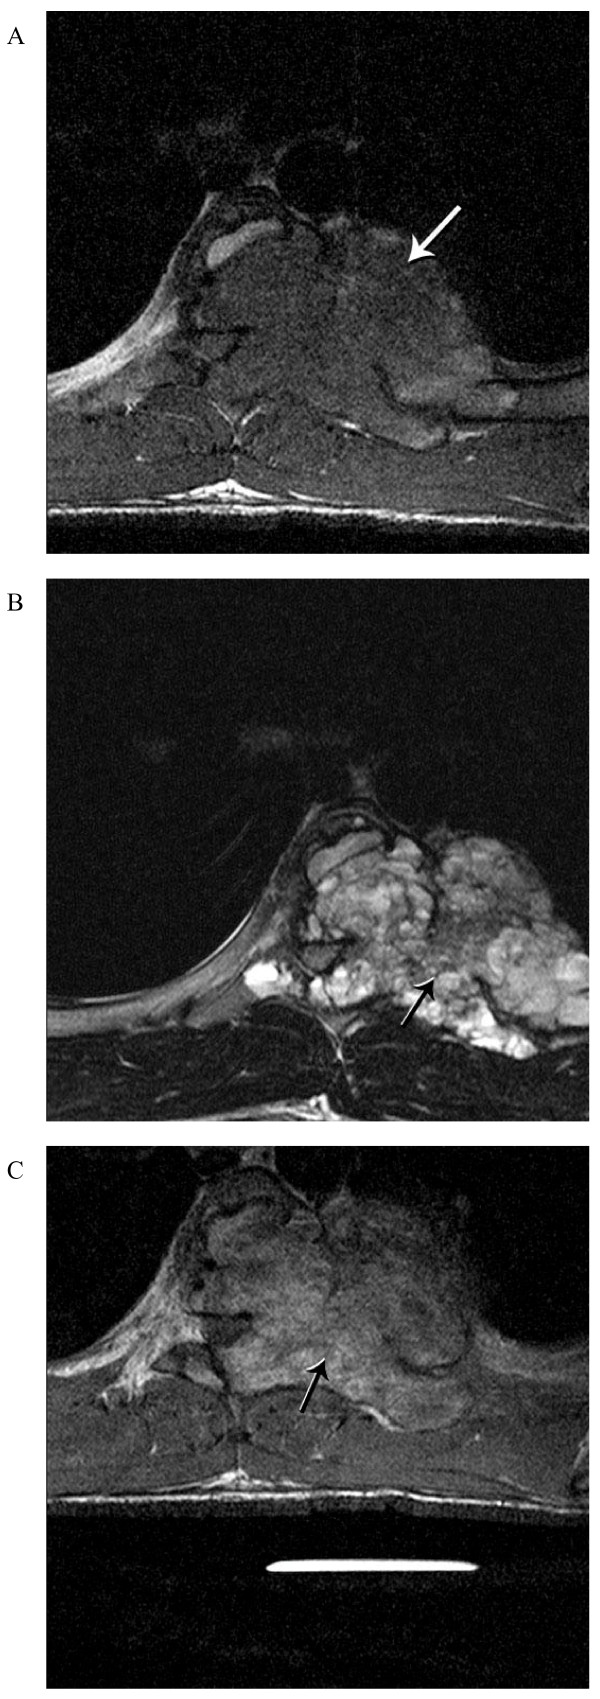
Pre-operative axial (A) T1-weighted and (B) T2-weighted MRI demonstrate a large heterogeneous low and high signal intensity mass lesion involving T6. (C) Enhanced T1-weighted MRI shows a more homogenous high signal T6 mass.
